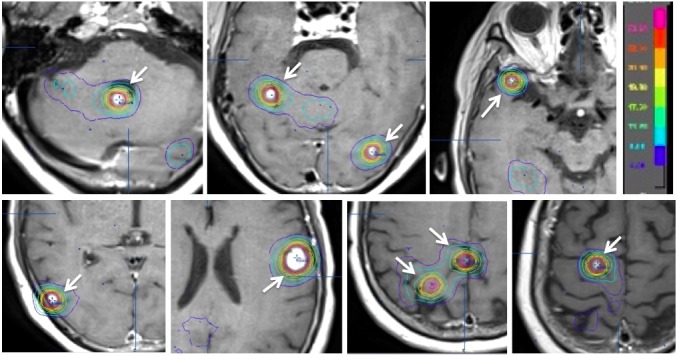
Pre-SRS MRI of brain lesions (Dose planning on iPlan) Axial MRI with Gd enhancement on iPlan (BrainLAB, Tokyo) radiation therapy planning system (RTPS) workstation. Nine small brain lesions (arrows) were treated by single session stereotactic radiosurgery (SRS). Each lesion was targeted with four arcs by dynamic conformal arc (DCA). 100% dose=22 Gy (single fraction SRS), D95=100%dose.
